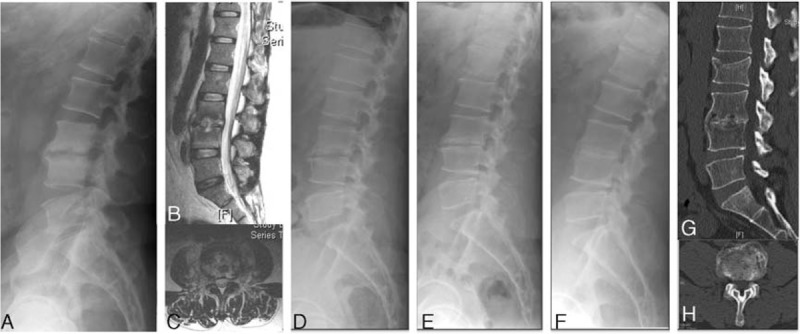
A 35 year-old man presented with severe pain in the lower back and leg, and was diagnosed with an L3–4 methicillin-resistant *Staphylococcus aureus* infection. (A–C) Preoperative plain radiograph and magnetic resonance imaging showed L3–4 disc and endplate destruction. (D) Plain radiograph at 1 month after surgery. (E) Plain radiograph at 1 year after treatment showed endplate sclerosis without further destruction. (F–H) Plain radiograph and computed tomography at 5 years after treatment showed well-maintained lumbar lordosis.
